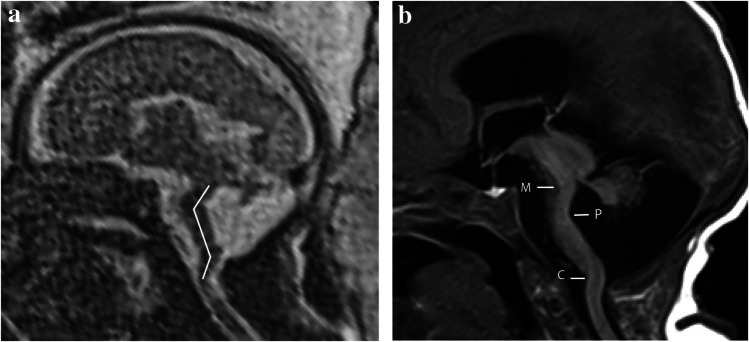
Examples of kinked brainstem. Fetal MRI (a) sagittal T2 SSFSE midline brain image at 26 2/7 weeks shows abnormal brainstem formation with kinked deformity and severe vermian hypoplasia. The suspected diagnosis was a tubulin mutation, although a genetic diagnosis was not made. Postnatal brain MRI of a different infant with Walker-Warburg (b) shows abnormal exaggeration of mesencephalic (M), pontine (P), and cervical (C) flexures of the brainstem on a sagittal T1-weighted image through the midline posterior fossa
